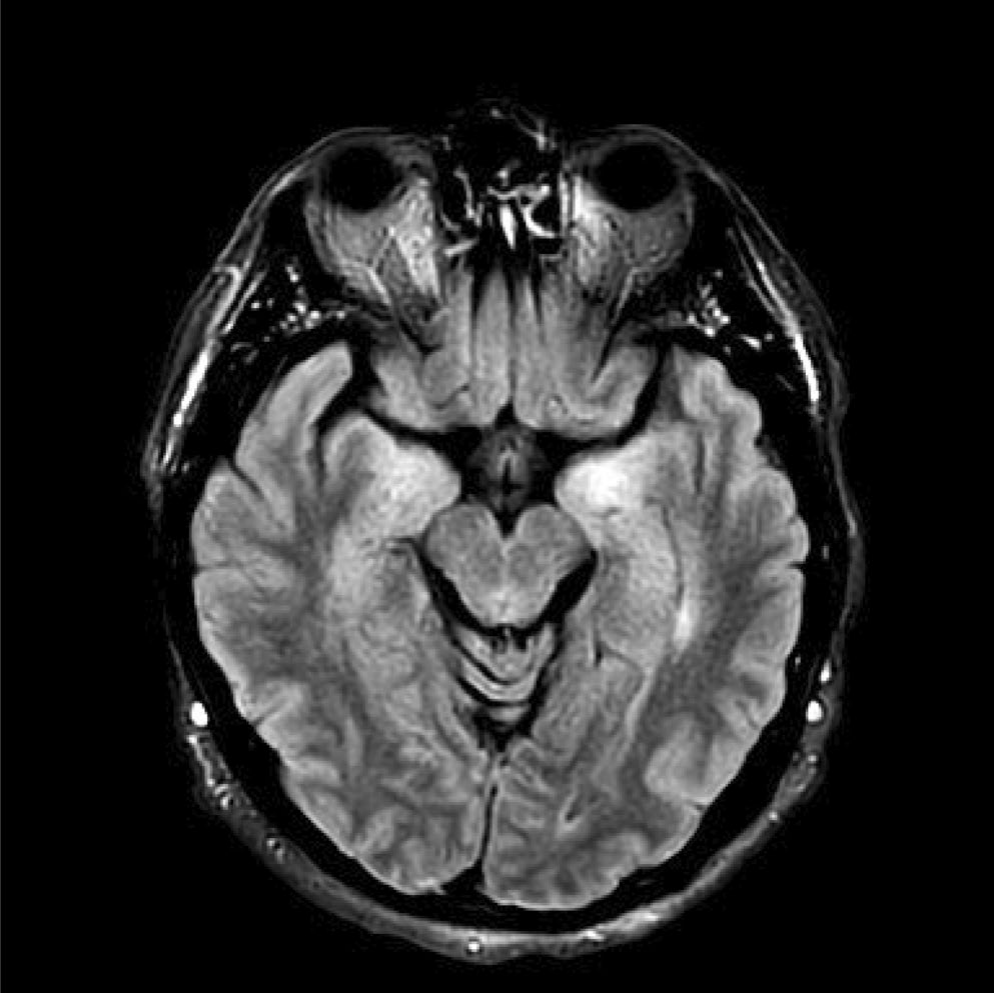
FIGURE 1 Brain RM T2‐FLAIR revealing hyperintensities in bilateral temporomesial regions.
